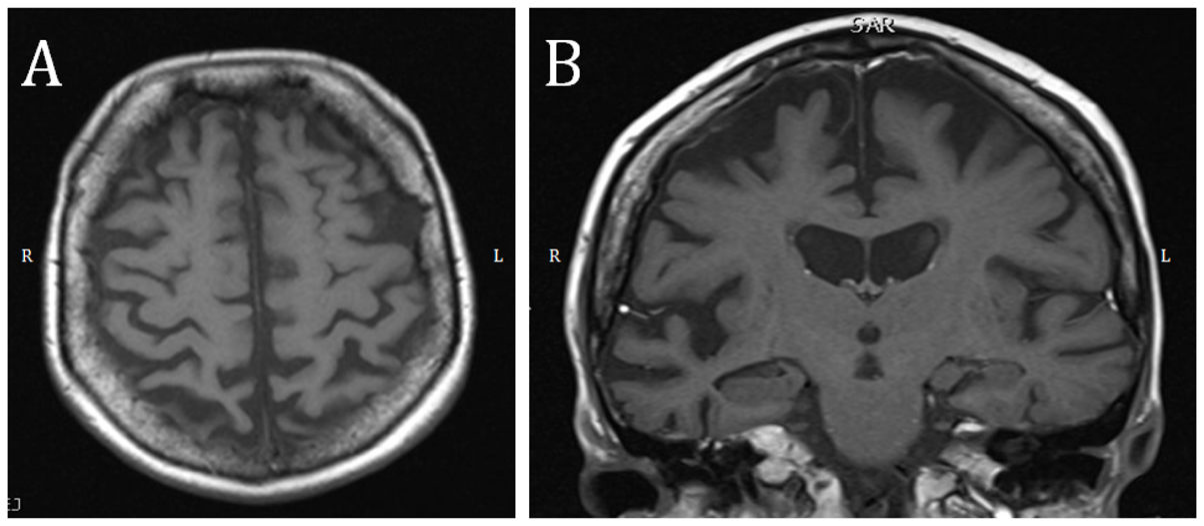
MRI of the brain. (A) Diffuse asymmetrical cortical atrophy (right>left) seen on an axial cut. The atrophy is prominent in the parietal lobes. (B) This coronal section shows that the asymmetrical cortical atrophy (right>left) extends to the posterior part of the superior temporal gyrus.
